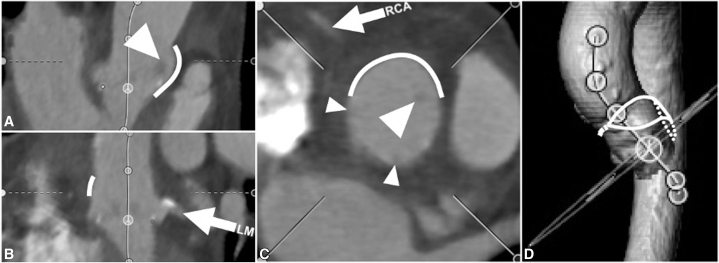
Representative appearance of computed tomography (CT) multiplanar reconstruction of the aortic root after left-right (LR) aortic root enlargement. Orthogonal planar views (A, B, C) show the left (LM) and right (RCA) coronary arteries (*arrows*), the LR valve strut (*large arrowheads*) well away from the patch (*thick line*) behind it, and the LN and RN struts adjacent to the aortic wall (*small arrowheads*). Blood flow entering the right sinus from above, where the patch has expanded the STJ (*thick line* in C), as well as blood flow passing behind the LR strut (between the *large arrowhead* and *thick line* in A and C) into the left sinus, appear to mitigate risk of sinus sequestration during future VinV TAVI. The 3-dimensional CT aortic reconstruction (D) shows the medial portion of the aortotomy (closed primarily) and the location of the patch (*solid* and *dotted outlines*) sewn into the middle and lateral portion of the curved aortotomy, spiraling down through the LR commissure. *VinV TAVI*, Valve-in-valve transcatheter aortic valve implantation.
